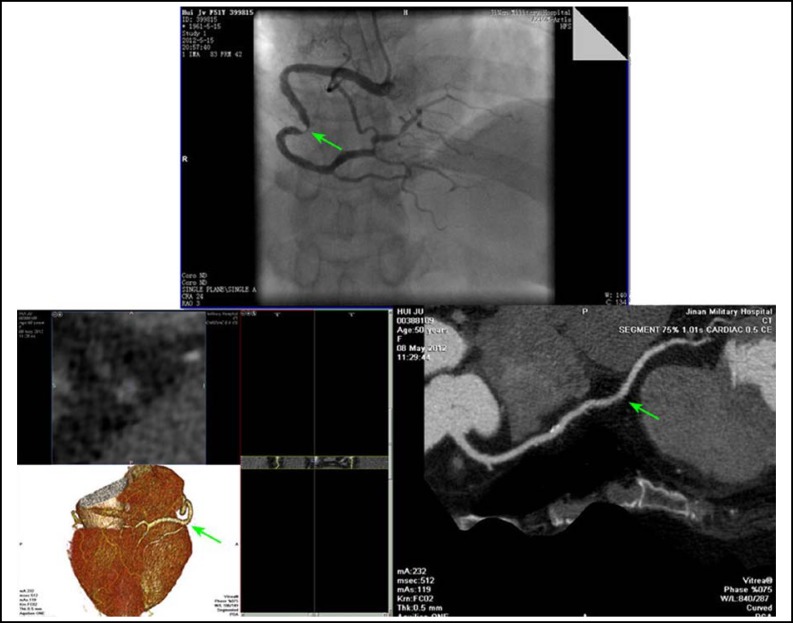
Tortuous lesion effected judgment of coronary stenosis degree by CAG and CTA. CAG showed severe stenosis in the middle of RCA, CTA showed no obvious stenosis
